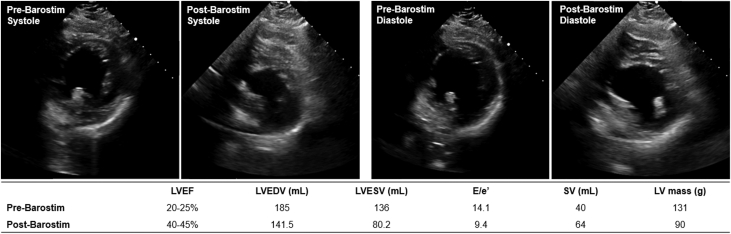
Case 1: Echocardiographic Images of Pre- and Post-Barostim Implant During Systole and Diastole With Associated Clinical Metrics of Interest
